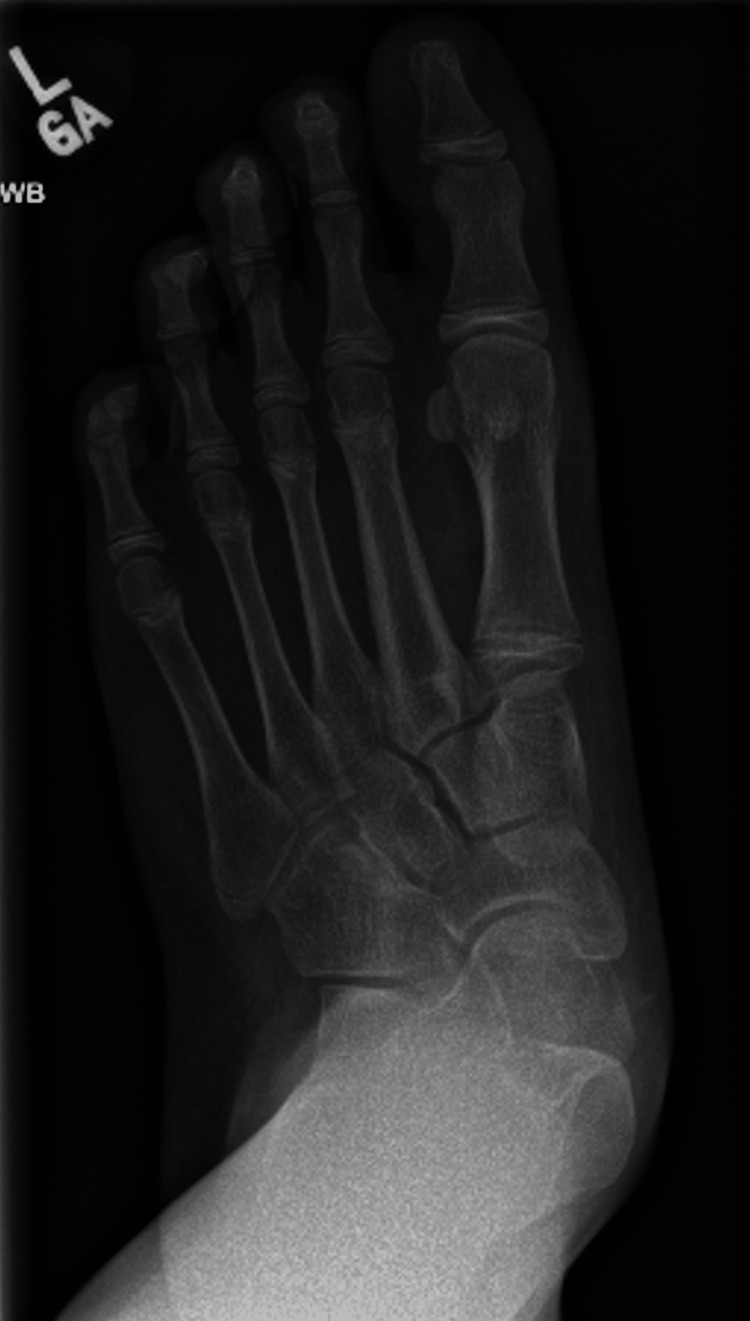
A pre-operative weight-bearing DP radiograph of the left foot, demonstrating increased talonavicular uncoverage and an increased Kite's angle DP: dorsoplantar
